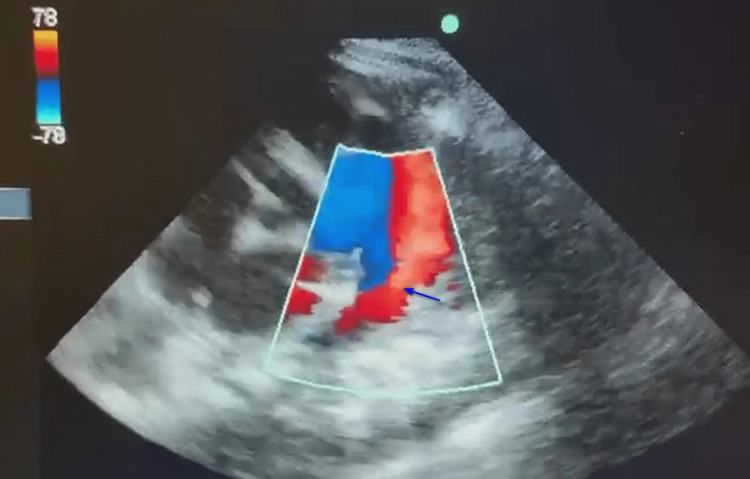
Color Doppler of the patent ductus arteriosus. Color Doppler of the patent ductus arteriosus showing predominantly flowing left to right shunt (only red flow), as seen in the parasternal short axis view marked by a deep blue arrow.
